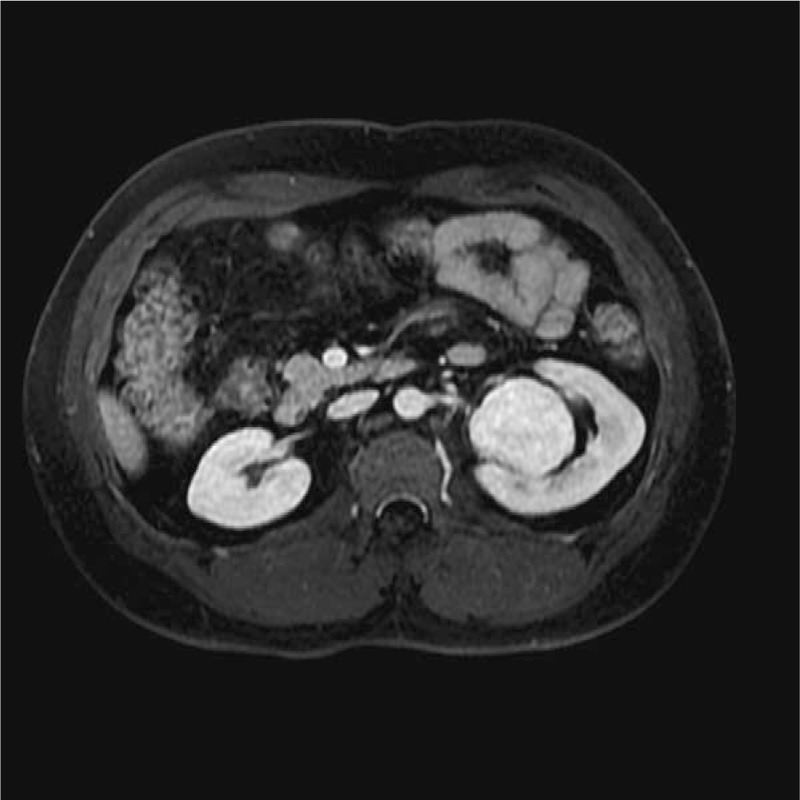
On dynamic contrast-enhanced magnetic resonance image, the venous phase image showed uniformly high enhancement.
